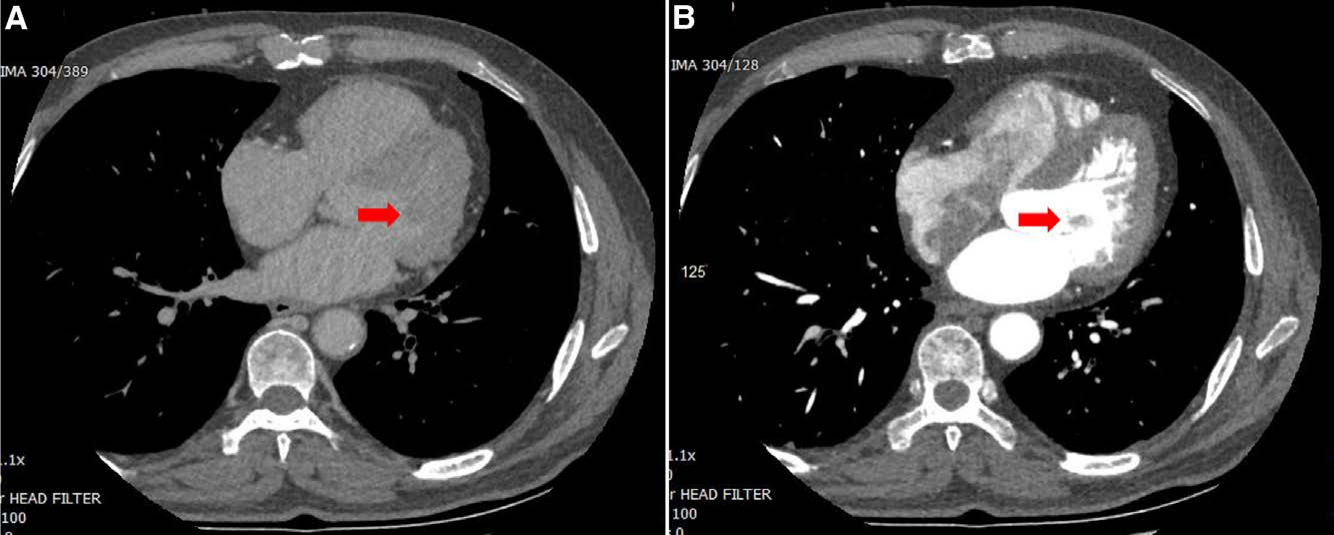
Noncontrast (A) and contrast-enhanced CT (B) imaging of the heart revealed an oval hypodense lesion (red arrow), which appeared to be attached to the anterior mitral valve leaflet by a small pedicle.
